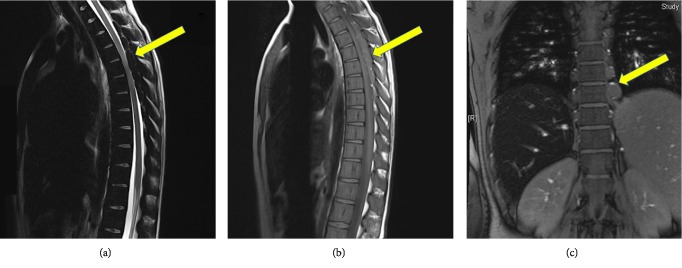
Follow up MRI of the spine after blood transfusion: (a) sagittal T2-weighted, (b) sagittal T1-weighted, (c) coronal T1-weighted images showing interval reduction in cord compression.
